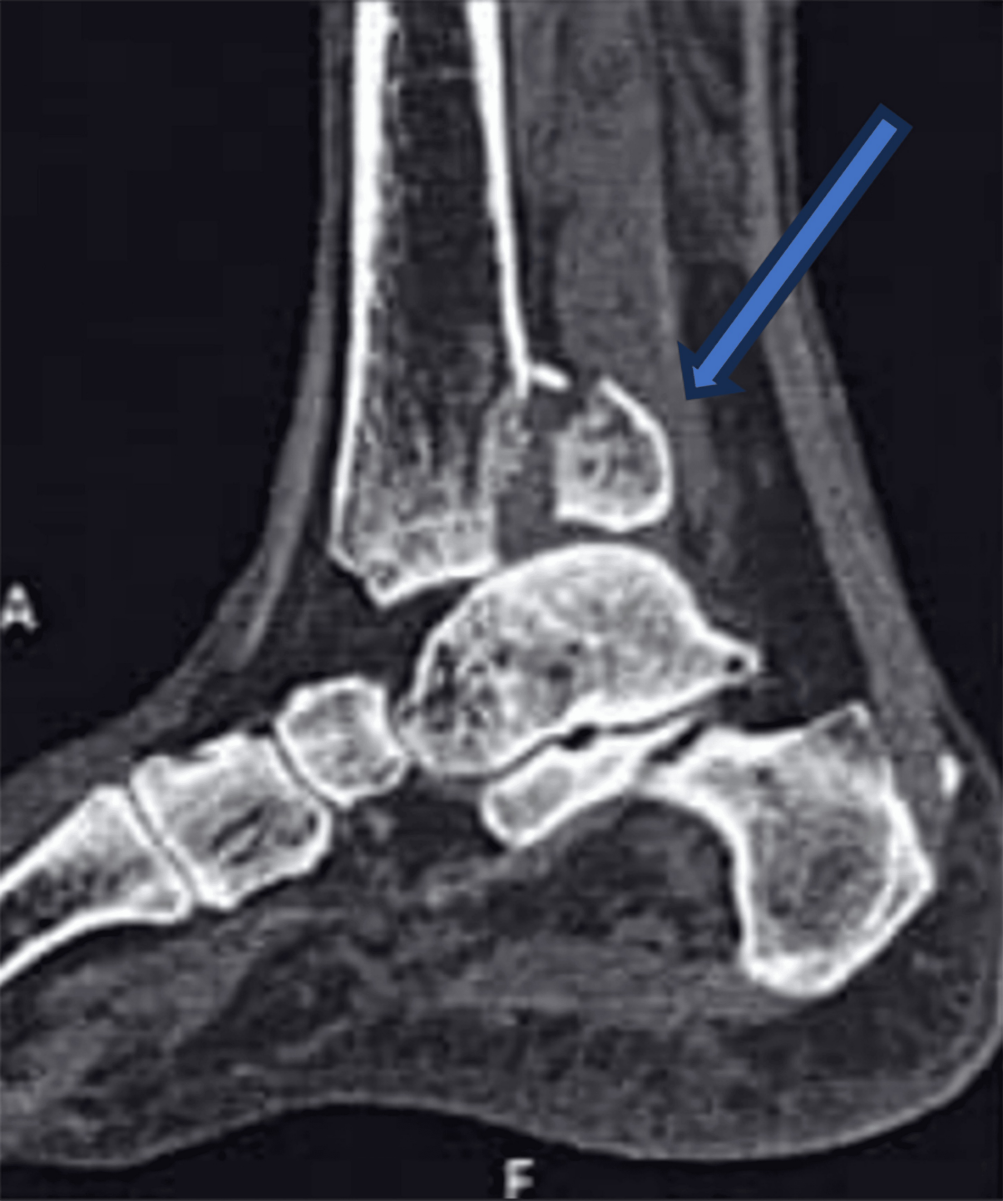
Sagittal section CT scan of an ankle A sagittal section of the CT scan of the ankle showing posterior malleolus fracture. CT: Computed tomography
